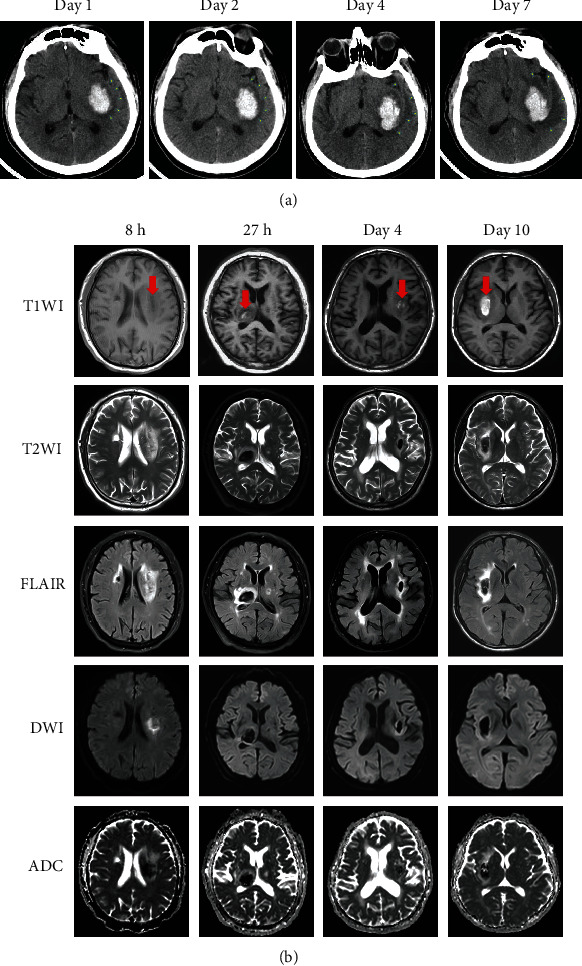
The imaging characteristics of PHE at different stages after ICH. (a) Cranial CT images on days 1, 2, 4, and 7 after acute ICH in a patient. PHE (a rim around the hematoma indicated by yellow arrows) was not prominent on day 1 after ICH and gradually increased from days 1 to 7 after ICH. (b) MRI characteristics of PHE at 8 hours, 27 hours, day 4, and day 10, respectively, after the onset of symptoms in 4 patients with ICH. The red arrows in the T1WI images indicate the location of the hematoma. The signal characteristics of the hematomas changed over time in the T1WI and T2WI images after ICH, with PHE presented as a thin or wide rim with a strong signal in the T2WI and FLAIR images in the areas surrounding the hematoma. A strong signal on the DWI image but a weak signal on the ADC map appeared in the perihematomal area 8 hours after ICH. It may represent the appearance of ionic edema or cytotoxic edema in the perihematomal area. However, typical imaging characteristics of vasogenic edema in the perihematomal region were observed at 27 hours, day 4, and day 10 after the onset of the symptom. Vasogenic edema presented as a normal signal on the DWI images, and a strong signal surrounds the hematoma on the ADC map at 27 hours, day 4, and day 10 after ICH in 3 patients. Abbreviations: CT: computed tomography; PHE: perihematomal edema; ICH: intracerebral hemorrhage; T1WI: T1-weighted MRI; T2WI: T2-weighted MRI; FLAIR: fluid-attenuated inversion recovery; DWI: diffusion-weighted imaging; ADC: apparent diffusion coefficient.
